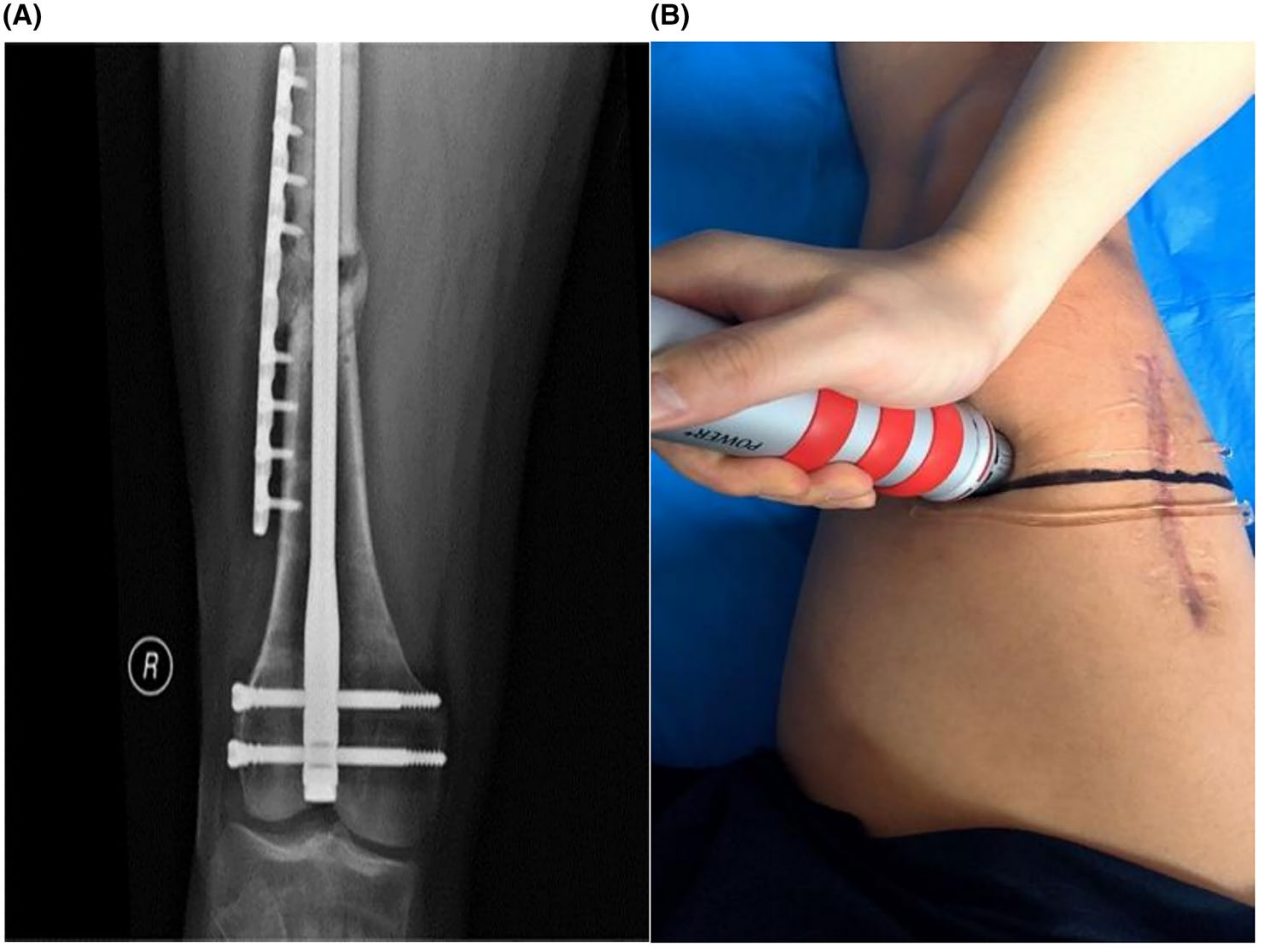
(A) A follow‐up X‐ray 1 month later showed partial callus formation. (B) The positioning approach was modified to ultrasound‐guided localization of all fracture ends for shockwave application.
